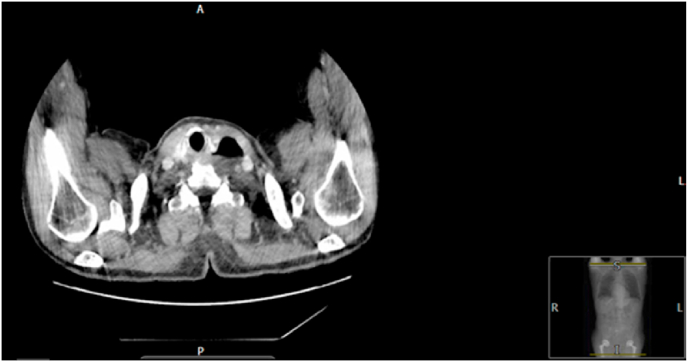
CT Contrast Neck, Left Pharyngeal pouch.
